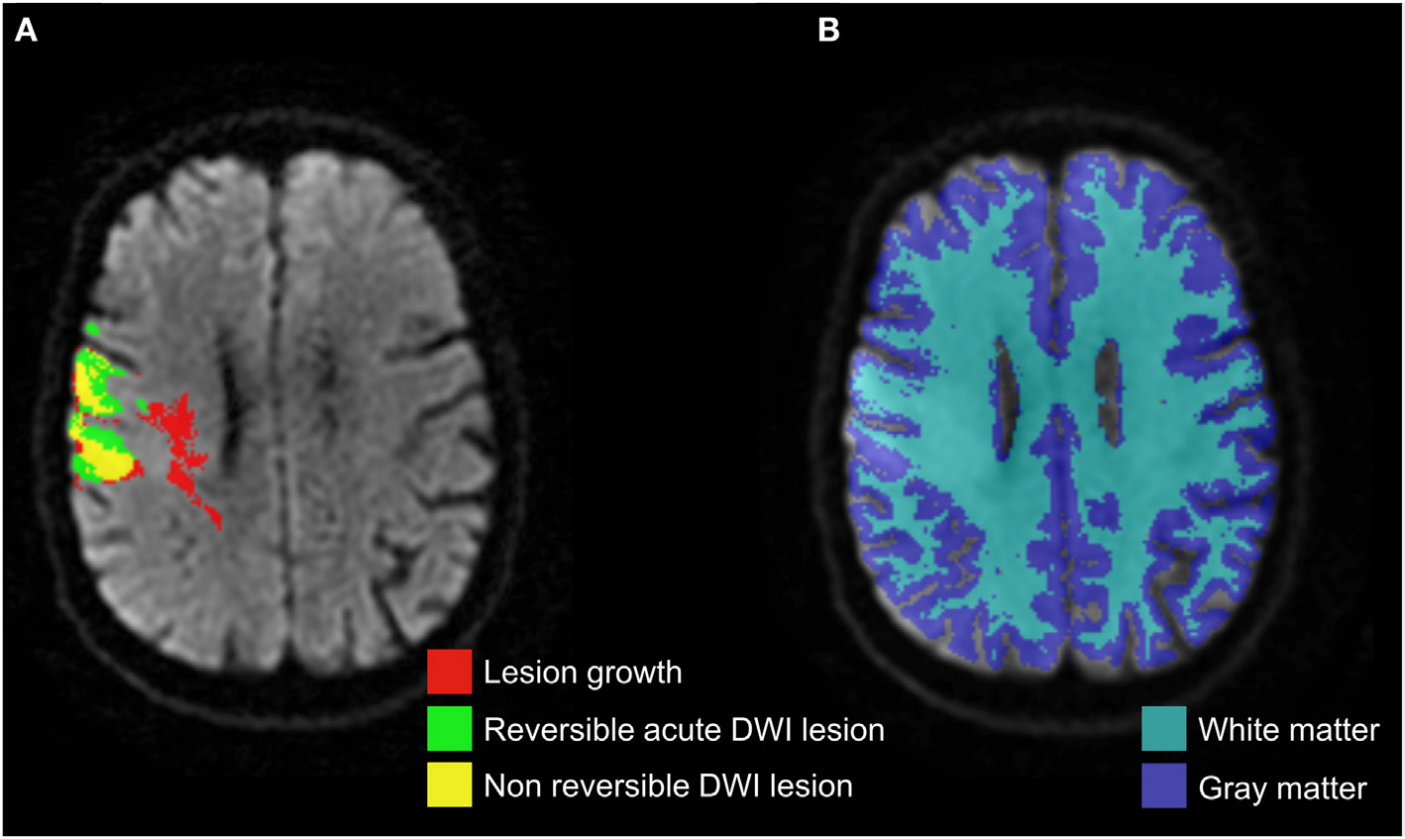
Co-registered masks of acute diffusion-weighted imaging (DWI) lesion, final infarct, and corresponding lesion growth (A) overlaid on acute DWI, which were then segmented by the gray matter and white matter probabilistic maps (B).
